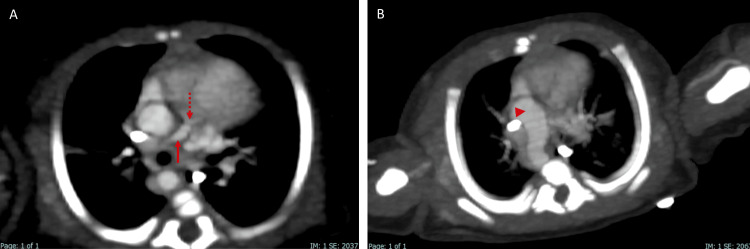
Cardiac CT angiography in oblique axial at the level of the heart. (A) The image shows the right ventricular outflow tract with hypoplasia of the pulmonary artery (arrow) and pulmonary atresia (dashed arrow). (B) Additionally, the right-sided aortic arch (arrowhead) is observed.
